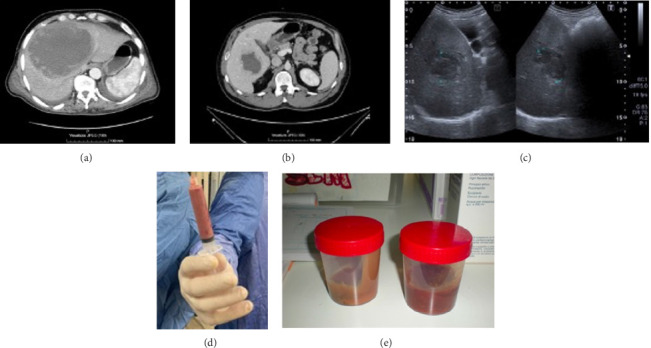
Appearance of the amoebic abscess of Case 1 (a) and Case 2 (b) on the CT scan. (c) Appearance of the amoebic abscess of Case 2 on ultrasound; “anchovy paste” appearance of the material drained from Case 1 (e) and Case 2 (d) abscesses.
